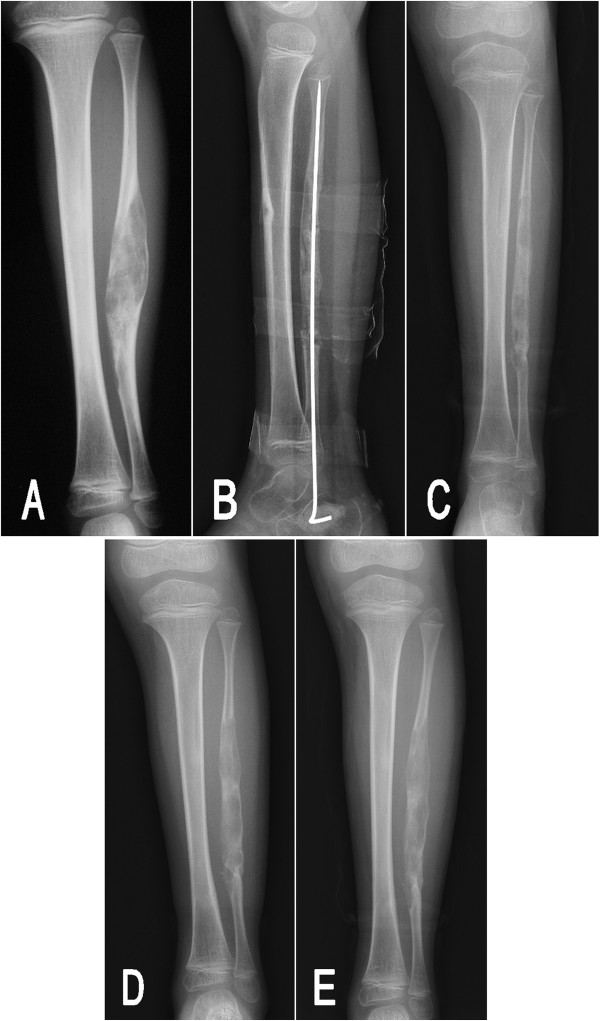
Preoperative and postoperative radiographs of patient 2. (A) A preoperative radiograph indicated an expansile, multiseptated lucent lesion in the fibula. (B) A good union and alignment had been obtained at 8 weeks postoperation. (C) At 4.5 months follow-up after surgery, the lesion recurred with multiple small irregular lucencies. At postoperative 2 years (D) and 4.5 years (E) of follow-up, these small lucencies gradually fused into a long, expansile lesion and appeared progressive over time.
